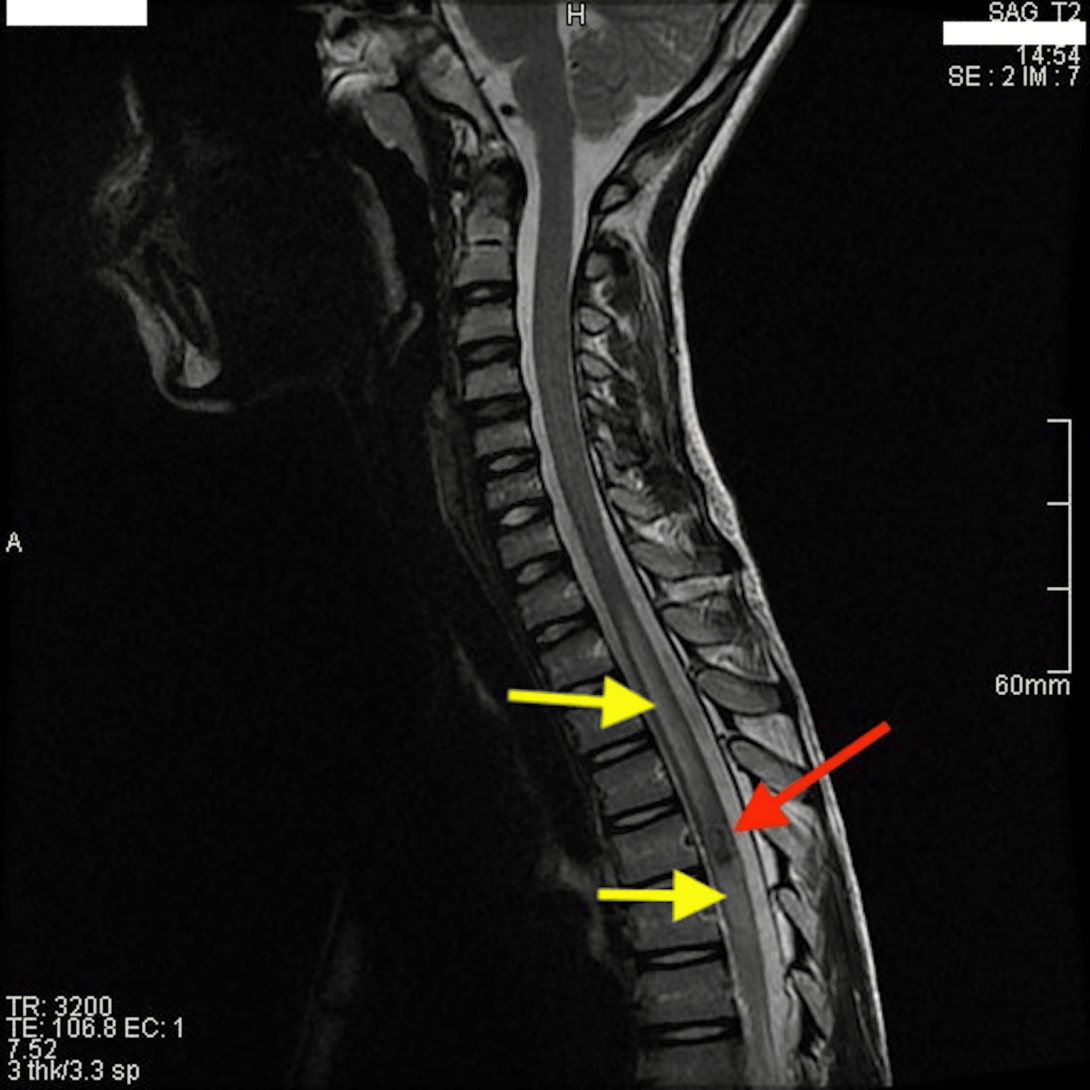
Intramedullary cavernous malformation on T2-weighted imaging. Sagittal T2-weighted MRI demonstrating a T5 cavernoma. Note the heterogeneous mixed signal intensity of the cavernous (red arrow) and the edematous perilesional reaction (yellow arrows).
